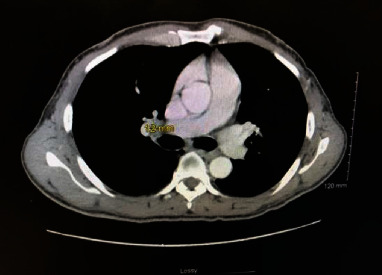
CT chest with contrast imaging of right hilar mass.
